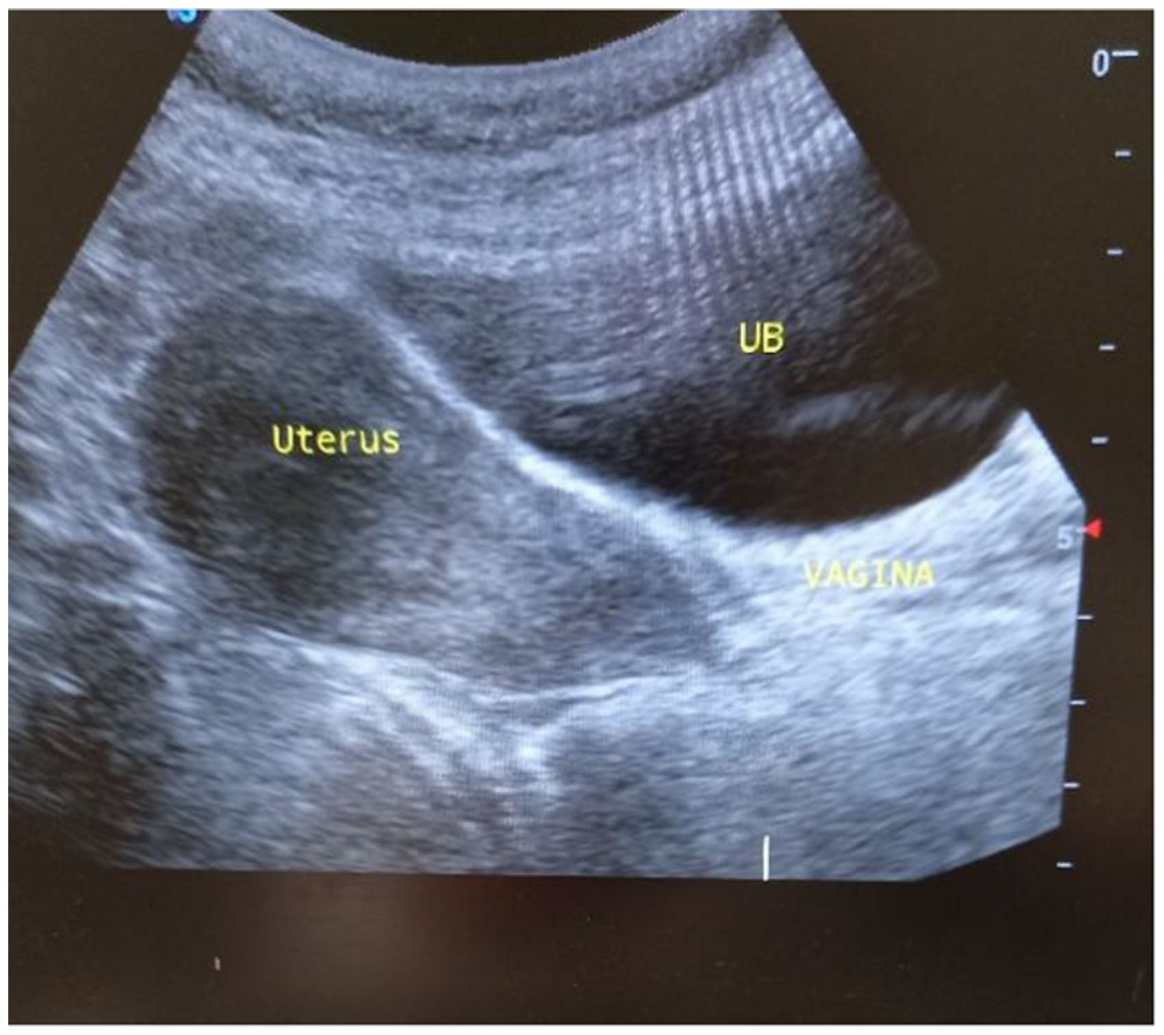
Pelvic ultrasound showing a sagittal view of a normal uterine and cervical outline, UB, Urinary bladder.
